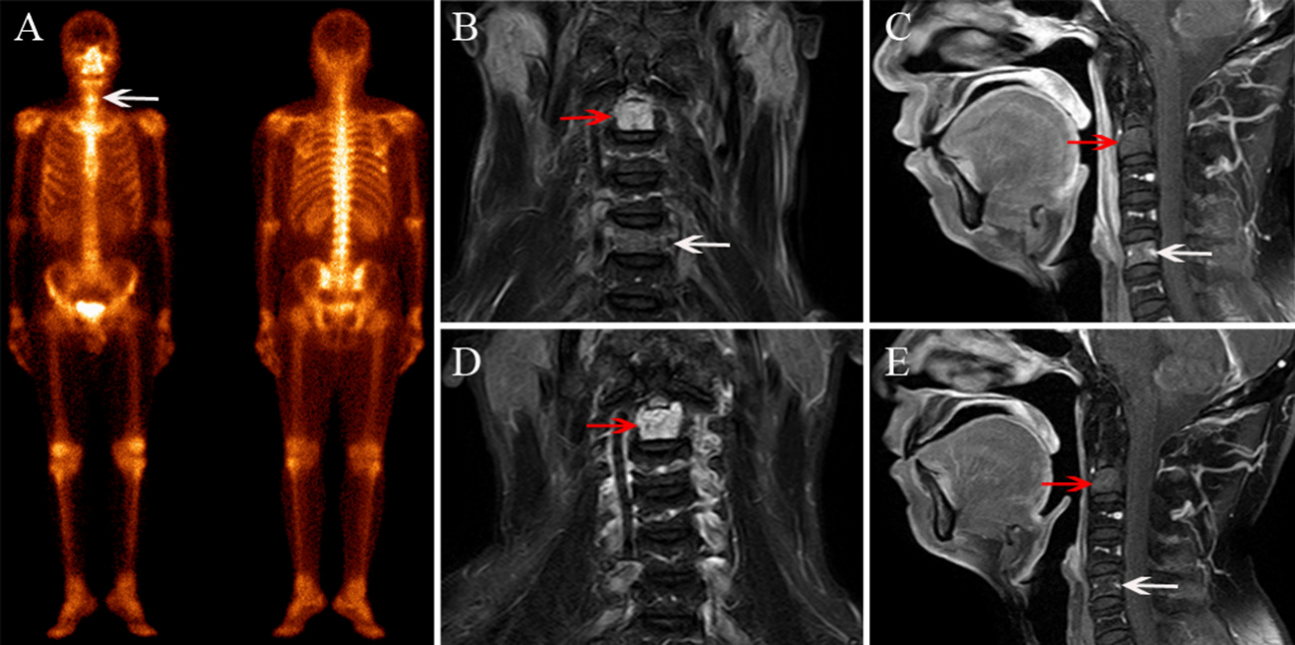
A 42-year–old male diagnosed with cervical spine ORN after RT for NPC. (A) BS shows increased radiotracer uptake in C5 (white arrow) and the seventh right rib. (B) Coronal FS T2–weighted image shows hyperintensity in the C2 (white arrow) and C5 vertebra (red arrow). (C) Sagittal contrast-enhanced T1-weighted image shows mild enhancement in C2 and moderate enhancement in C5. (D,E) MRI follow-up examination after 8 months, coronal FS T2-weighted image (D), and sagittal contrast-enhanced T1-weighted image (E) show that the signal change in C2 has remained stationary and the abnormal signal in C5 has disappeared.
